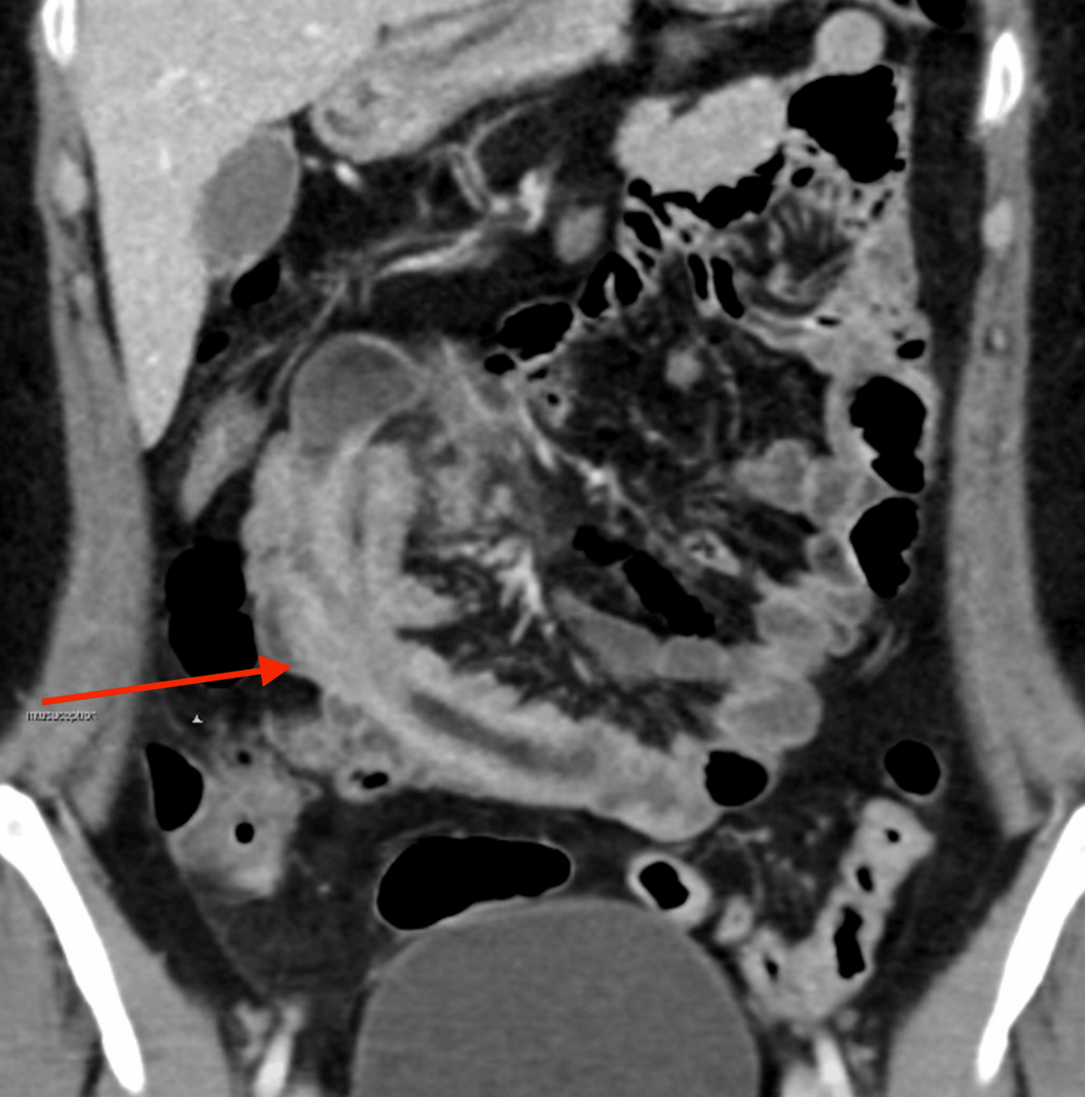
Coronal CT abdomen after contrast showing intussusception with a lead point (red arrow)
